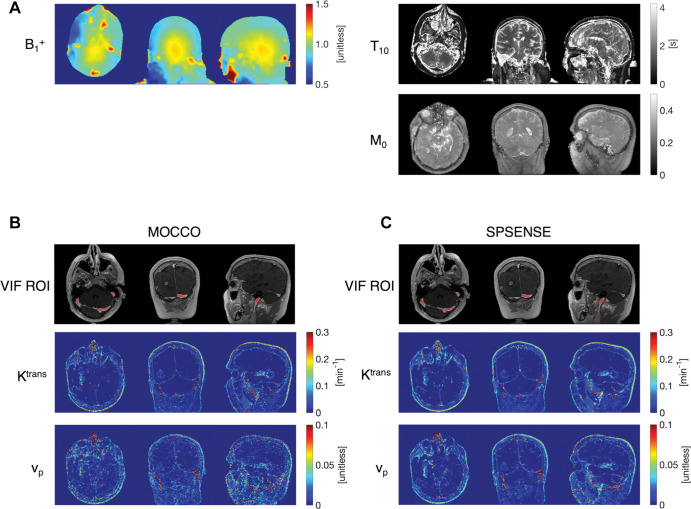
Representative parameter maps of dynamic contrast-enhanced MRI examination in a 59-year-old woman in axial, coronal, and sagittal views for model consistency constrained (MOCCO) and sparse sensitivity encoding (SPSENSE) frameworks. *A,* Baseline maps for radiofrequency inhomogeneity (B_1_+), baseline T1, and proton density map M_0_. *B, C,* Images obtained after administration of contrast agent with regions of interest for vascular input functions on internal jugular veins, transverse and sigmoid dural venous sinuses (red), plasma volume fraction (v_p_), and volume transfer constant (K^trans^) for MOCCO and SPSENSE, respectively.
